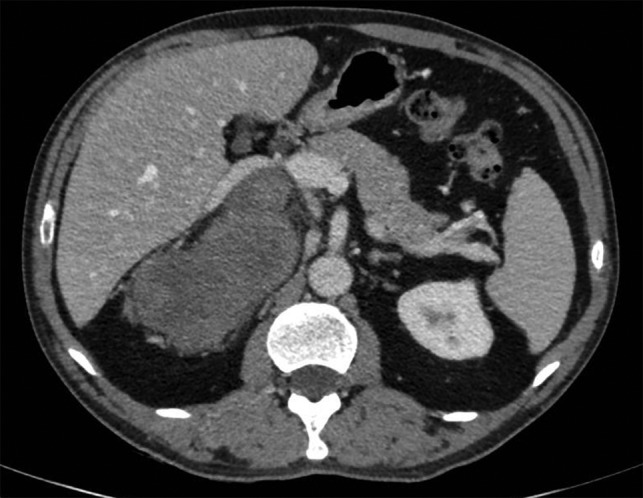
CT imaging of the lesion.
